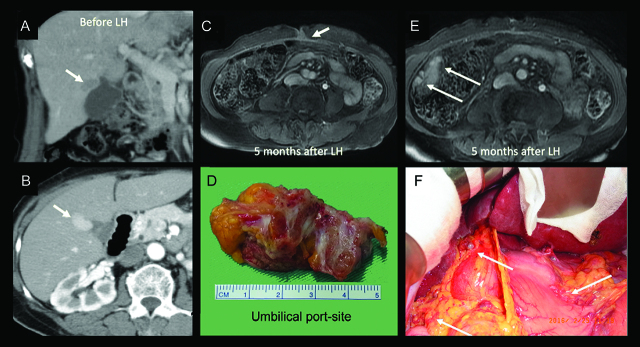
Patient #3 tumor location and late port site, subhepatic metastases. Results for patient #3, who had undergone laparoscopic hepatectomy (LH) for a 2.5 cm HCC in S5 (A, B); MRI imaging conducted within 5 months postoperatively and operative findings showed umbilical port site metastasis (C, D) and multiple nodules (1 cm in size) in the right subhepatic region (arrows) (E, F).
